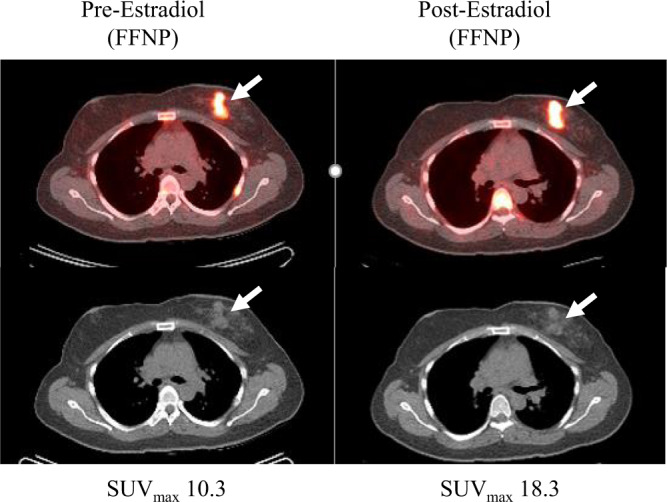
Responder. A woman with newly diagnosed ER+/PR+/HER2− invasive ductal carcinoma with metastatic disease at diagnosis in left axillary lymph nodes and a rib. She was treated with an aromatase inhibitor and palbociclib after the estradiol challenge test and had marked improvement of all lesions. Selected fused transaxial 21-[^18^F]fluorofuranyl-norprogesterone [FFNP]-PET/CT (top) and CT (bottom) images at baseline show intense FFNP uptake in the primary left breast cancer (arrows). One day after estradiol, tumor FFNP uptake measured as the maximum standardized uptake value (SUV_max_) (arrows) was increased by 78% from baseline.
